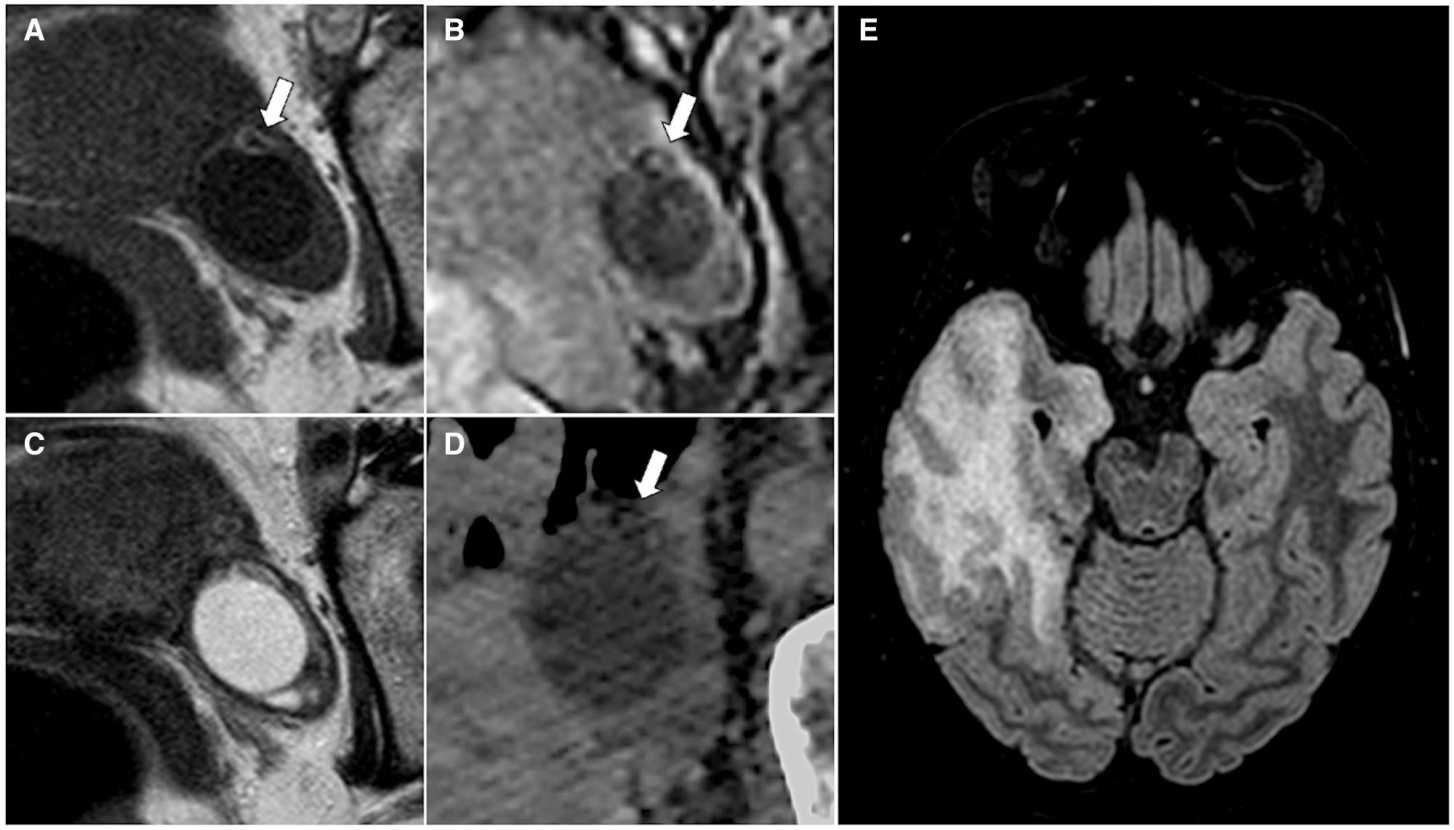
Lipid-poor mature cystic teratoma. Pelvic MRI (A-C) and CT (D) in a 30-year-old patient with a small left adnexal lesion. The fat component is confined to the cystic wall and shows high SI on T1-WI (arrow in A), low SI on T1-WI with fat saturation (B) and hypodense appearance on CT image (D). The patient underwent an MRI brain because presenting with confusion, amnesia, loss of consciousness and seizures. FLAIR sequence (E) shows oedematous changes in the right temporal lobe consistent with the diagnosis of anti-NMDA encephalitis. Abbreviations: SI = signal intensity; WI = weighted imaging.
